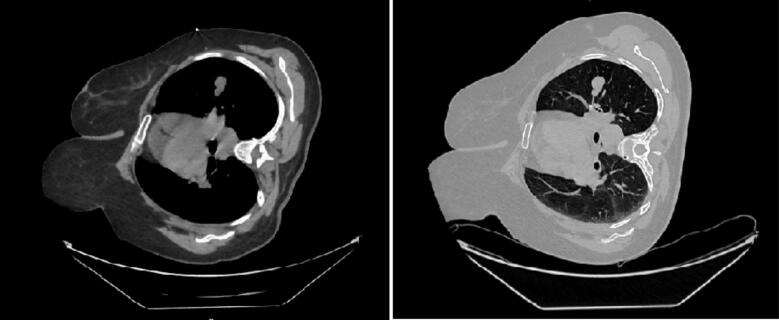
Chest spiral CT reveals a 17 × 25 mm lobulated mass in the left lung apex with no invasion into surrounding tissues.
